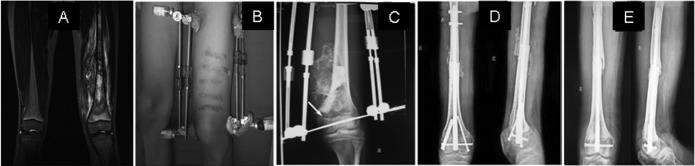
A patient who underwent epiphyseal preservation surgery. (A) A preoperative MRI image shows the tumor not reaching the physis. (B) An external fixator with a T-shaped piece for the epiphysis pins is attached for physeal distraction in the leg of a patient with malignant bone tumor. (C) An X-ray image shows the separation of the epiphysis from the metaphysis (white arrow). (D) An anteroposterior and lateral X-ray image shows the situation of a patient 6 months following tumor resection and reconstruction of the defect with allograft bone graft. (E) An anteroposterior and lateral X-ray image shows the situation of the same patient 2 years following tumor resection and reconstruction of the defect with allograft bone graft. MRI, magnetic resonance imaging.
